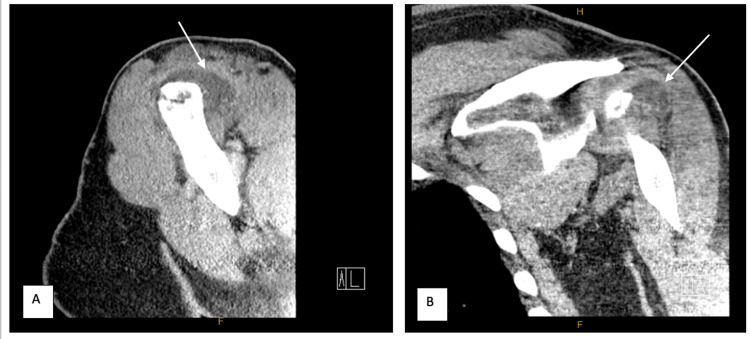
Axial (A) and coronal (B) CT with contrast images of the left shoulder demonstrating a distended subacromial bursa Arrows in A and B point to the distended subacromial bursa.
